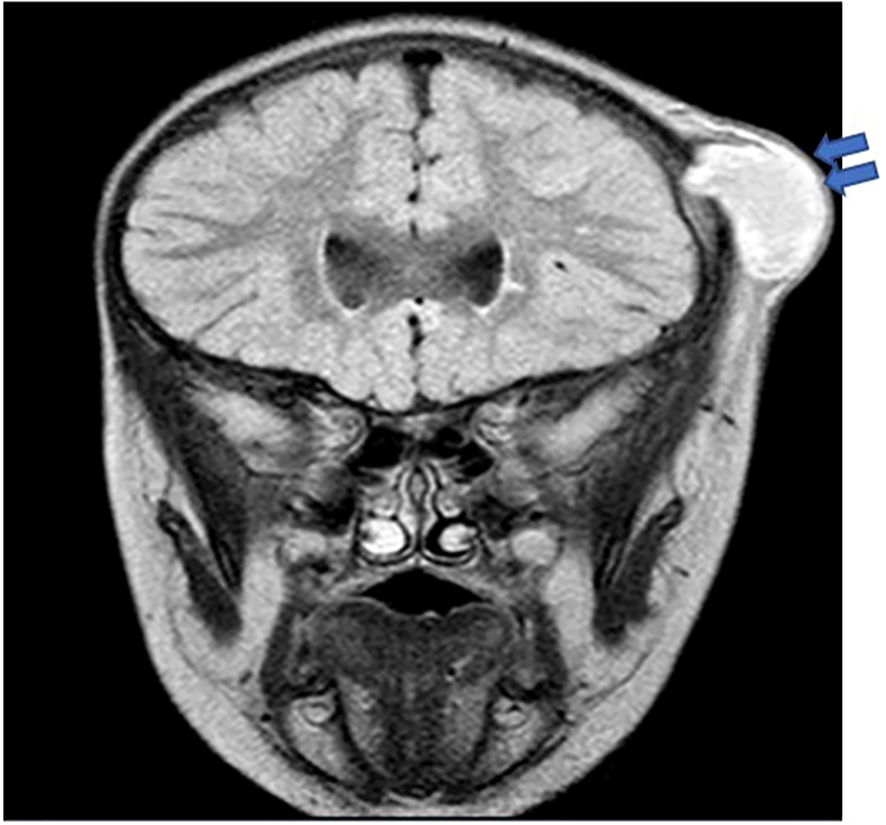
Brain MRI in P6 revealed a thick-walled cystic lesion (arrows) with some layering of signal arising from the left coronal suture with an underlying bony defect. No intracranial extension, although there is pachymeningeal enhancement underneath the lesion.
